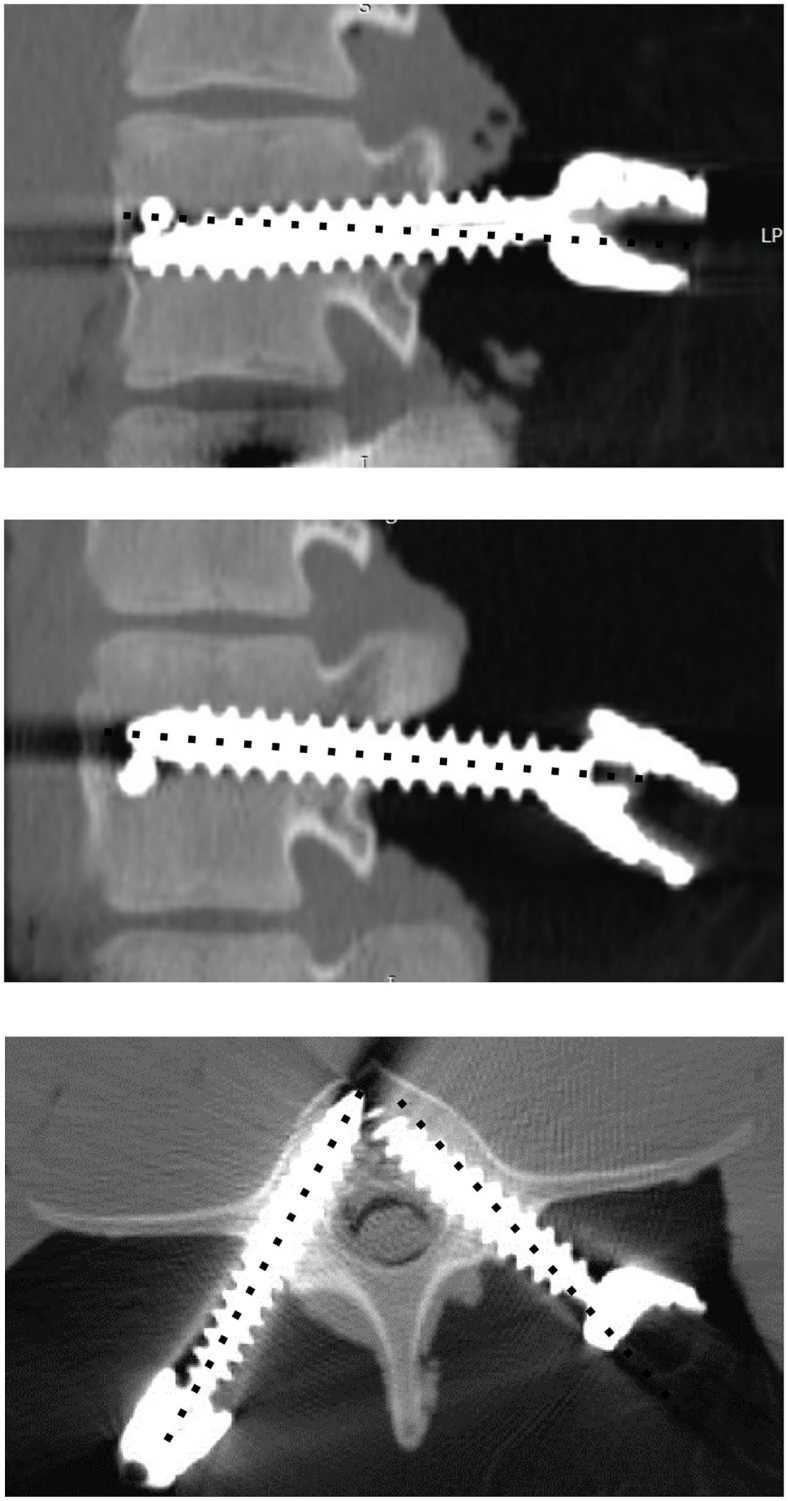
Parasagittal (upper and middle) and arbitrary axial planes (lower) obtained by postoperative computed tomography scans. The black dotted lines indicate the planned pedicle screw (PS) trajectories. The upper panel shows that the rostrocaudal angle of PS placed freehand caudally deviated relative to the planned angle, whereas the middle panel shows that the rostrocaudal angle of PS placed by perpendicular probing and screwing technique (PPST) was almost parallel to the planned angle. The lower panel shows that the mediolateral angle of the right PS placed by PPST was almost parallel to the planned angle, whereas the mediolateral angle of the left PS placed freehand medially deviated to the planned angle.
